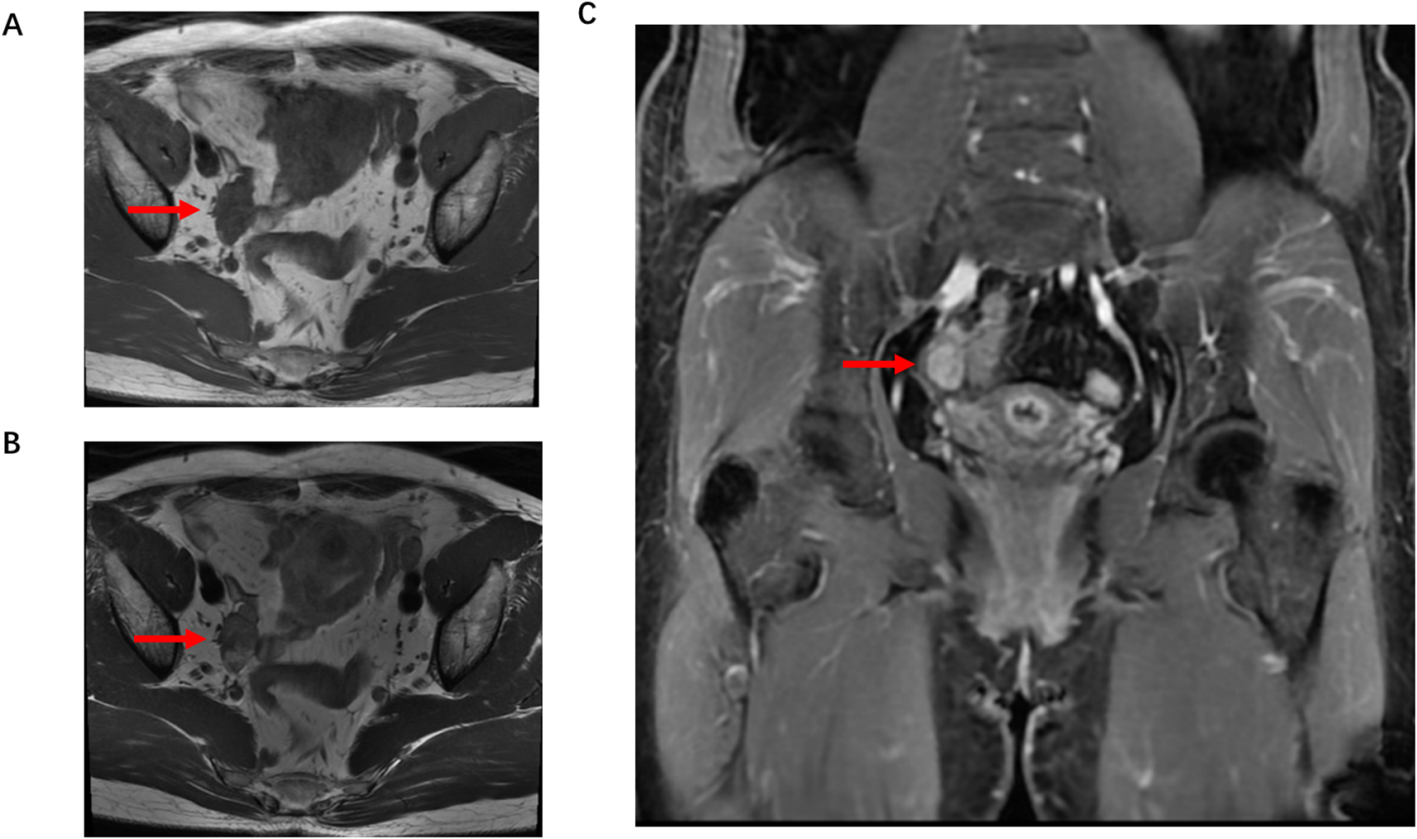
Pelvic contrast-enhanced magnetic resonance imaging (MRI) of the patient. (A) Axial T1-weighted image showed a nodular lesion in the right adnexa with a long T1 signal (arrow). (B) Axial T2-weighted image revealed a mixed T2 signal in the right adnexal nodule (arrow), measuring approximately 2.6×2.0 cm in size. (C) Coronal contrast-enhanced T1-weighted image demonstrated obvious enhancement of the right adnexal nodule (arrow), indicating increased vascularity. No abnormal signals or enhancing lesions were detected in the left adnexa. This imaging feature was highly suggestive of an ovarian neoplasm, which was later confirmed as AGCT.
